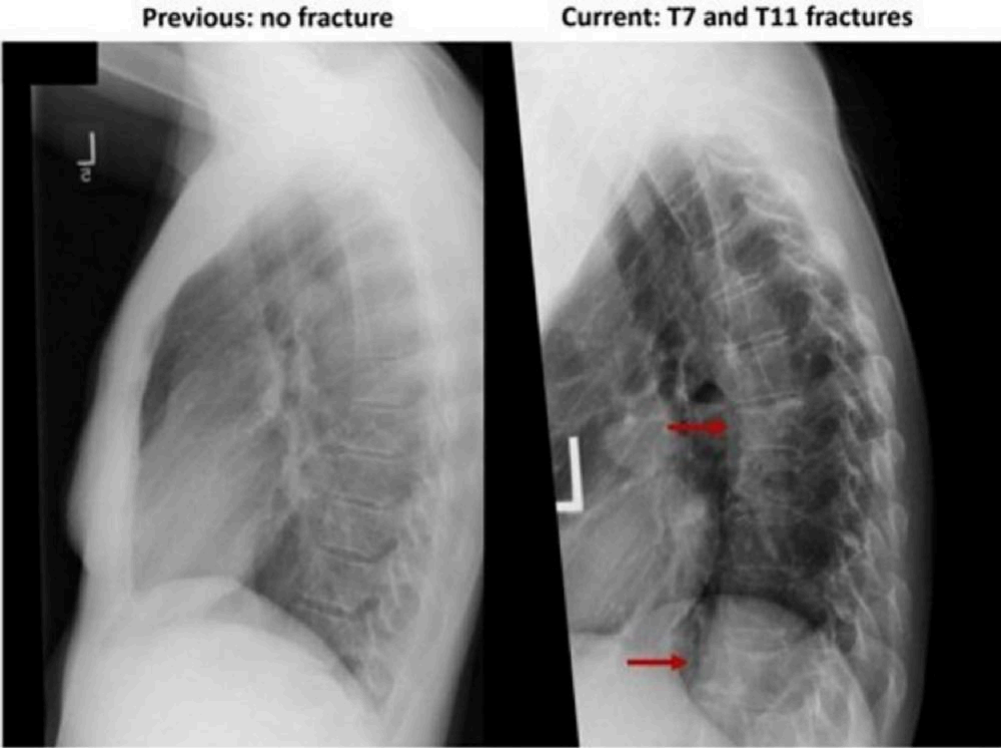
Thoracic spine X-rays, 13 years prior to and immediately following acute back pain.
